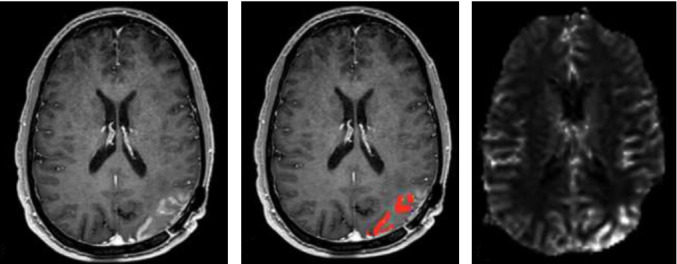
Brain magnetic resonance imaging perfusion (a) T1 postcontrast axial MRI showing nonspecific faint rim of peripheral ribbon like enhancement in the left parieto-occipital region. (b) MRI perfusion imaging showing increased cerebral blood flow in the left parieto-occipital region. (c) Increased cerebral blood volume in the left parieto-occipital region in a cortical pattern.
